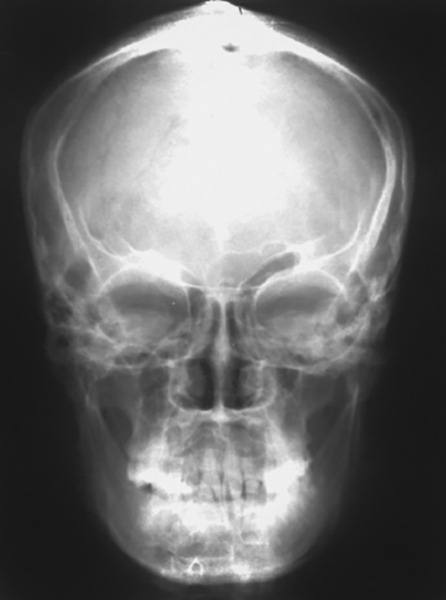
Posteroanterior (frontal) cephalometric radiograph
